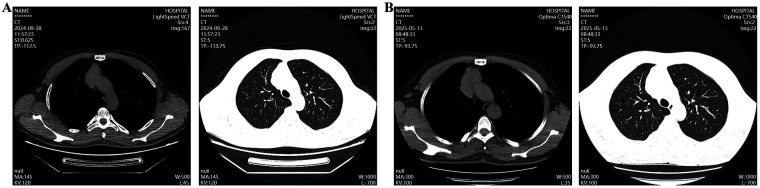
Chest CT scan revealed a mediastinal space-occupying lesion. (A) The first chest CT scan; (B) A chest CT scan after eight months.
